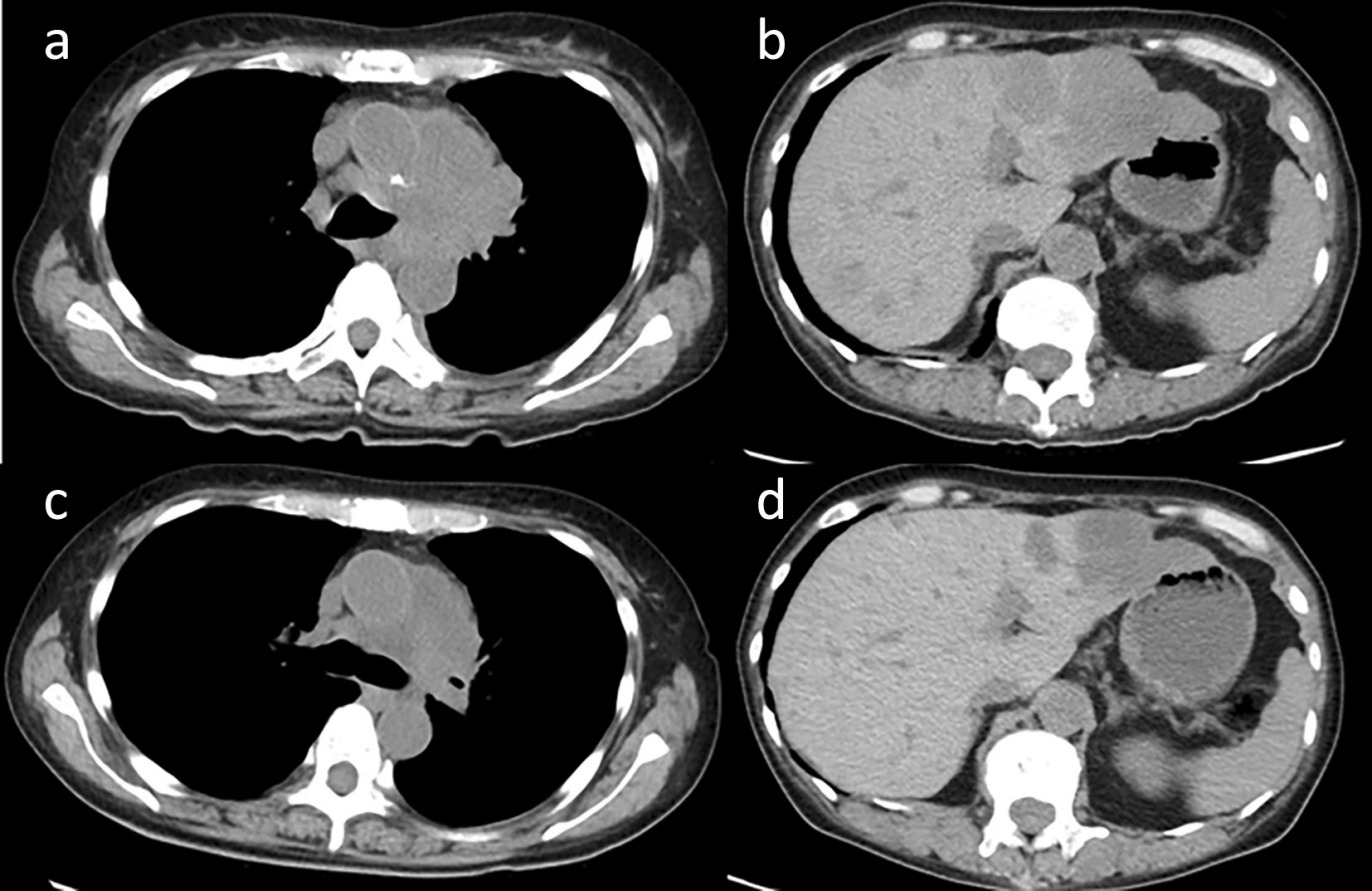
Chest computed tomography (CT) images before and after tarlatamab treatment. (a) Baseline CT demonstrating a mediastinal‐side mass in the left upper lobe. (b) Baseline CT demonstrating multiple hepatic metastases. (c) Day 13 post‐treatment: Marked reduction of the primary left upper lobe lesion. (d) Day 13 post‐treatment: Regression of hepatic metastases.
